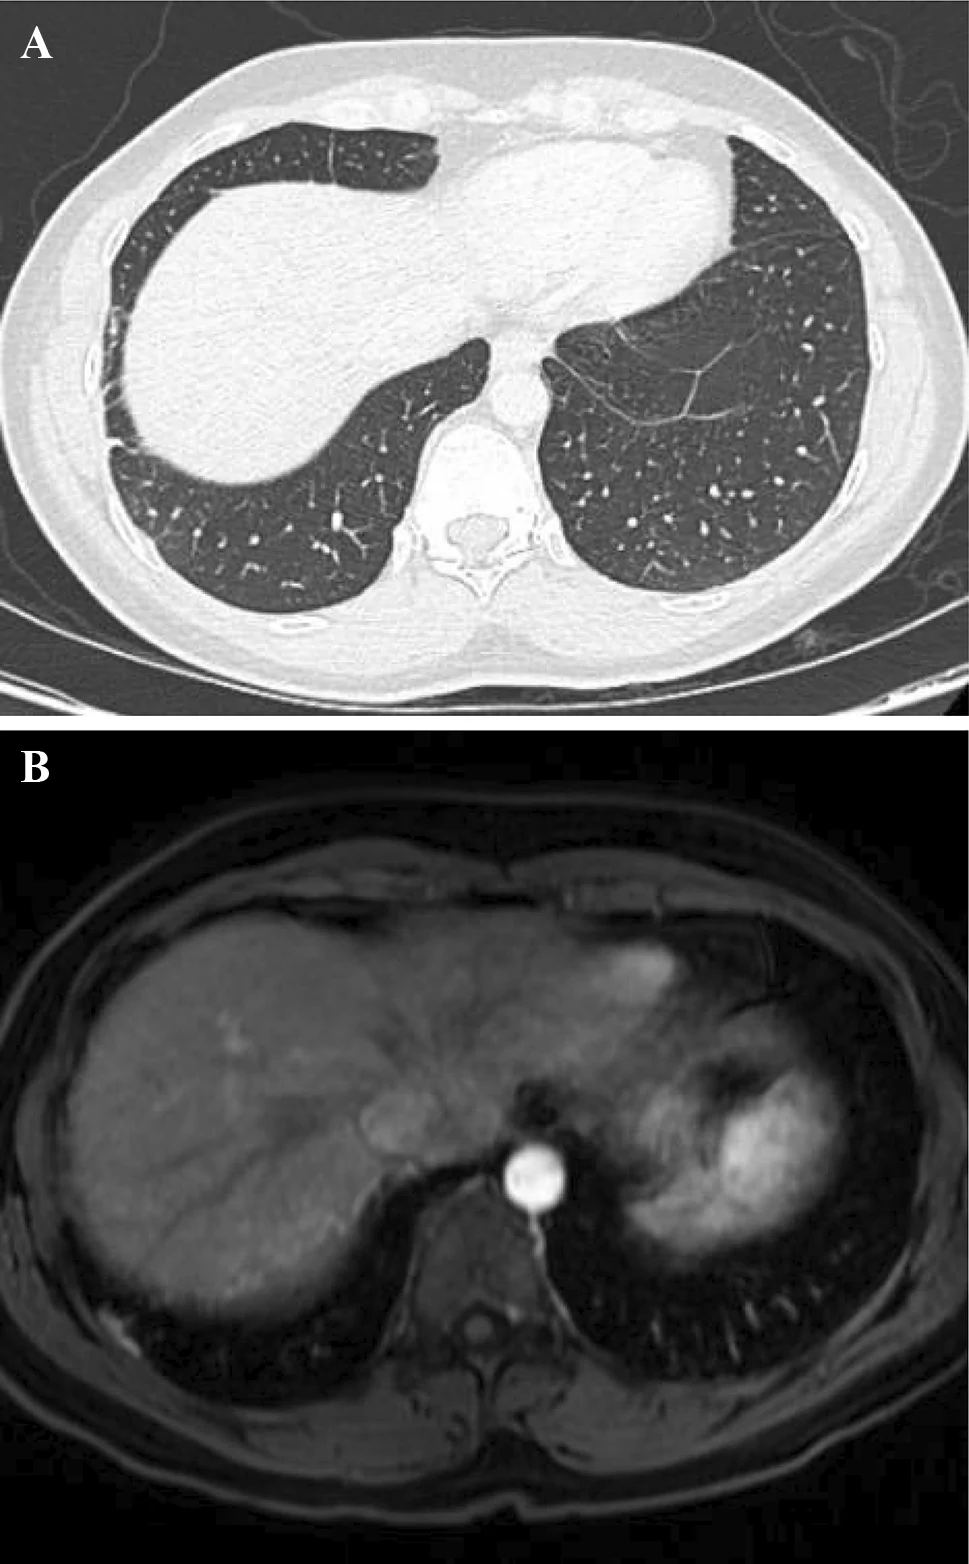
Computed tomography of chest [A] and magnetic resonance imaging of liver [B] with right pleural deposits and adhesions with atelectasis of the right lower lobe, possibly indicative of endometriosis and scarring on the diaphragm and/or the liver capsule, respectively
